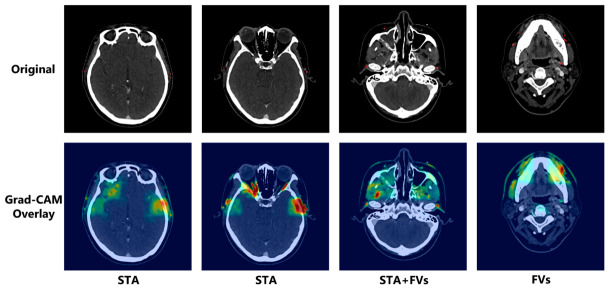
Grad-CAM visualization of the segmentation network under the proposed loss function. (Top row) Original craniofacial CTA slices and (bottom row) corresponding Grad-CAM activation overlay maps.
